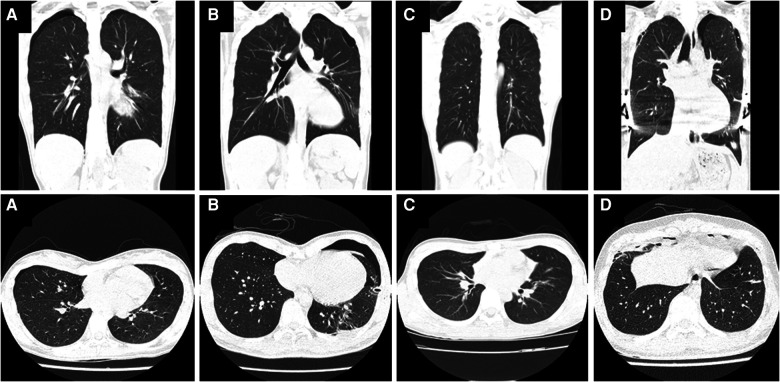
Chest computed tomography (CT) images showing primary spontaneous pneumothorax (PSP) in patients with pectus excavatum (PE). (A,a) Patient 1: An 18-year-old female with pneumothorax found incidentally during PE evaluation. (B,b) Patient 2: A 21-year-old male with PE found during evaluation and treatment of PSP. (C,c) Patient 4: A 15-year-old male with confirmed PE whose PSP occurred before the Nuss procedure. (D,d) Patient 5: An 18-year-old male with bilateral PSP unrelated to the surgery after a Nuss procedure to correct PE.
